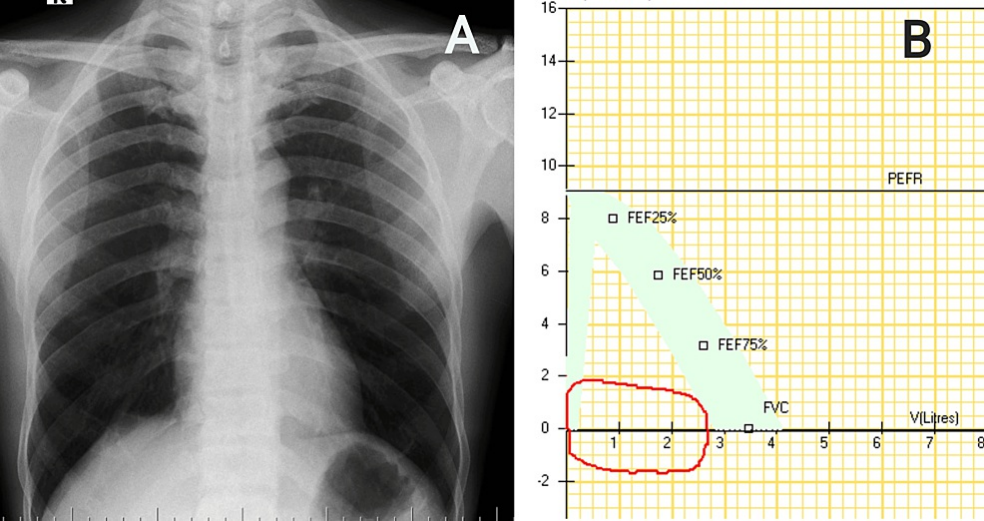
A) Chest radiograph PA view showing hyperinflated lung fields. B) Spirometry revealed flattening of the inspiratory and expiratory graph, suggestive of fixed airway obstruction with forced expiratory time of 1.8 seconds PA, posterior-anterior
